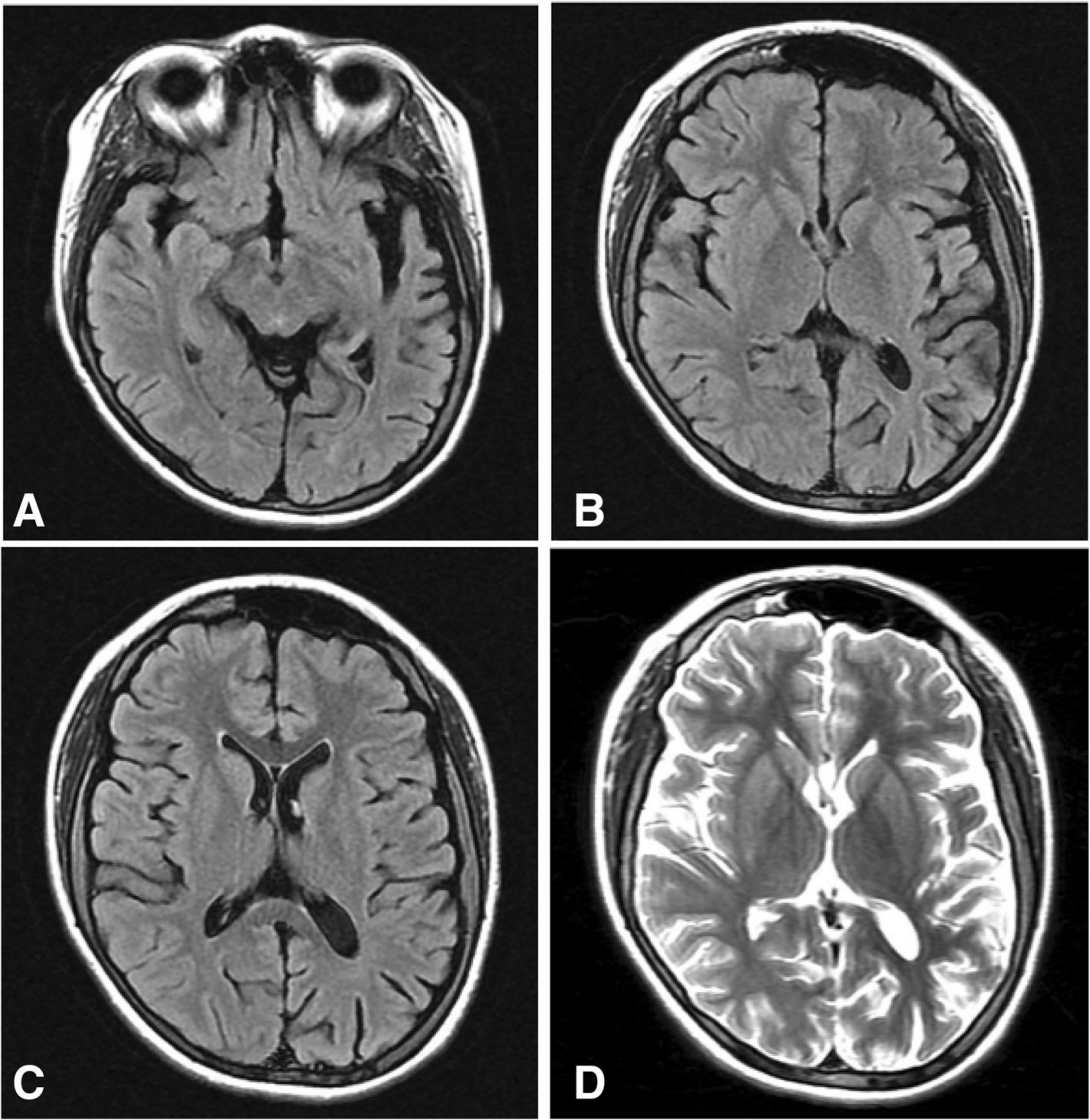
Brain magnetic resonance imaging. a–c Axial T2-weighted fluid-attenuated inversion recovery images showing left cerebral hemisphere cortical atrophy, with ipsilateral skull thickening and hyperpneumatization of the left frontal sinus, along with enlargement of the atrium, frontal, temporal, and posterior horns of the left lateral ventricle. Widening of sulci, cisternal spaces, and subarachnoid space is also noted. d Axial T2 weighted image showing the same description mentioned above
